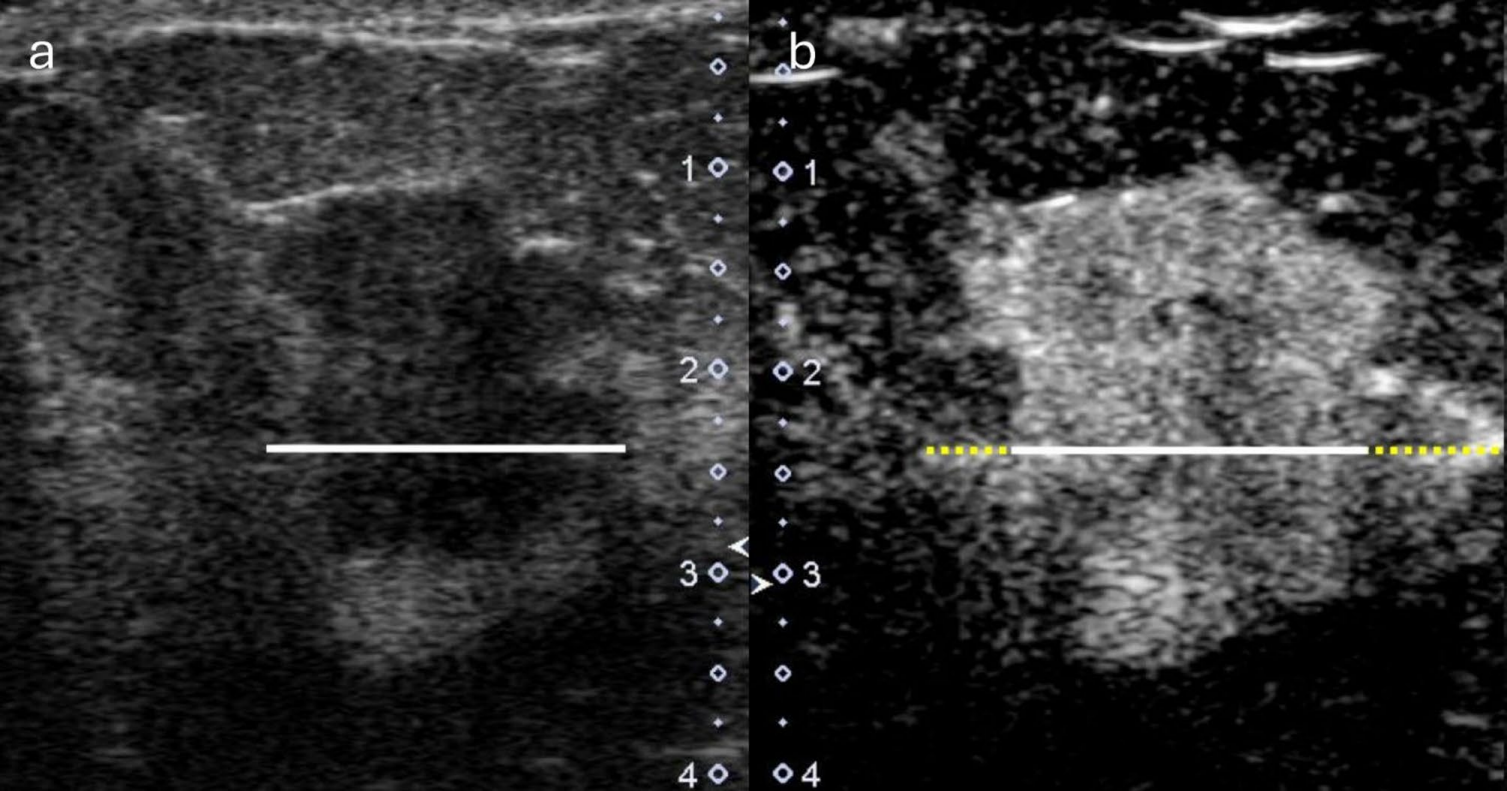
Representative images of patients grouped into DISCR. The patients with axillary node metastasis- positive breast cancer, who were a 65-year-old female (a) Diameter measurements by conventional ultrasonography, (b) diameter measurements by contrast-enhanced ultrasonography. The white solid line indicates the tumor size evaluated by conventional ultrasonography (21 mm). The yellow dashed line indicates the size discrepancy (6 mm). Because the discrepancy is ≥ 4 mm, this patient is categorized as DISCR
